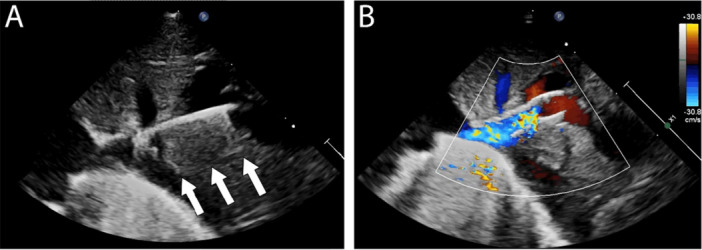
(A) Placement of the stent within the ductus venosus (longitudinal view of the upper abdomen). Prior to inflation, the position of the stent can be determined by its posterior echo‐extinction (arrows). (B) Color Doppler echocardiography after balloon inflation shows restored flow across the stented DV. [Color figure can be viewed at wileyonlinelibrary.com]
